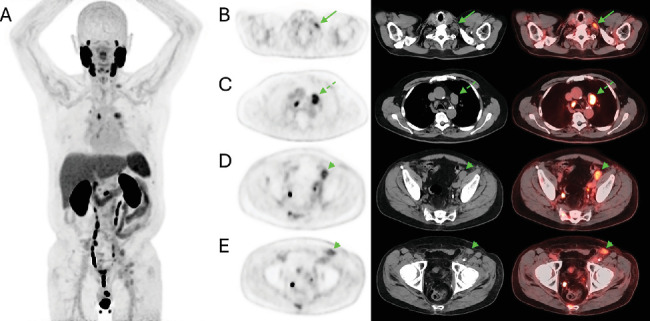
Maximum intensity projection (MIP) from a PSMA PET/CT study (A) performed for restaging of prostate cancer. Multiple new sites of PSMA-avid disease were detected including a left supraclavicular lymph node (*arrow*, B) measuring 19 × 13 mm with SUVmax 4.0. Mediastinal lymphadenopathy was present, including a subaortic lymph node (*dotted arrow*, C) measuring 33 × 21 mm with SUVmax 6.3. In addition, PSMA activity was found to localize to a left external iliac lymph node (*arrowhead*, D) measuring 25 × 17 mm with SUVmax 4.4. A left inguinal lymph node (*arrowhead*, E), later found to represent biopsy-proven metastatic MCC, measured 22 × 14 mm with SUVmax 3.4.
